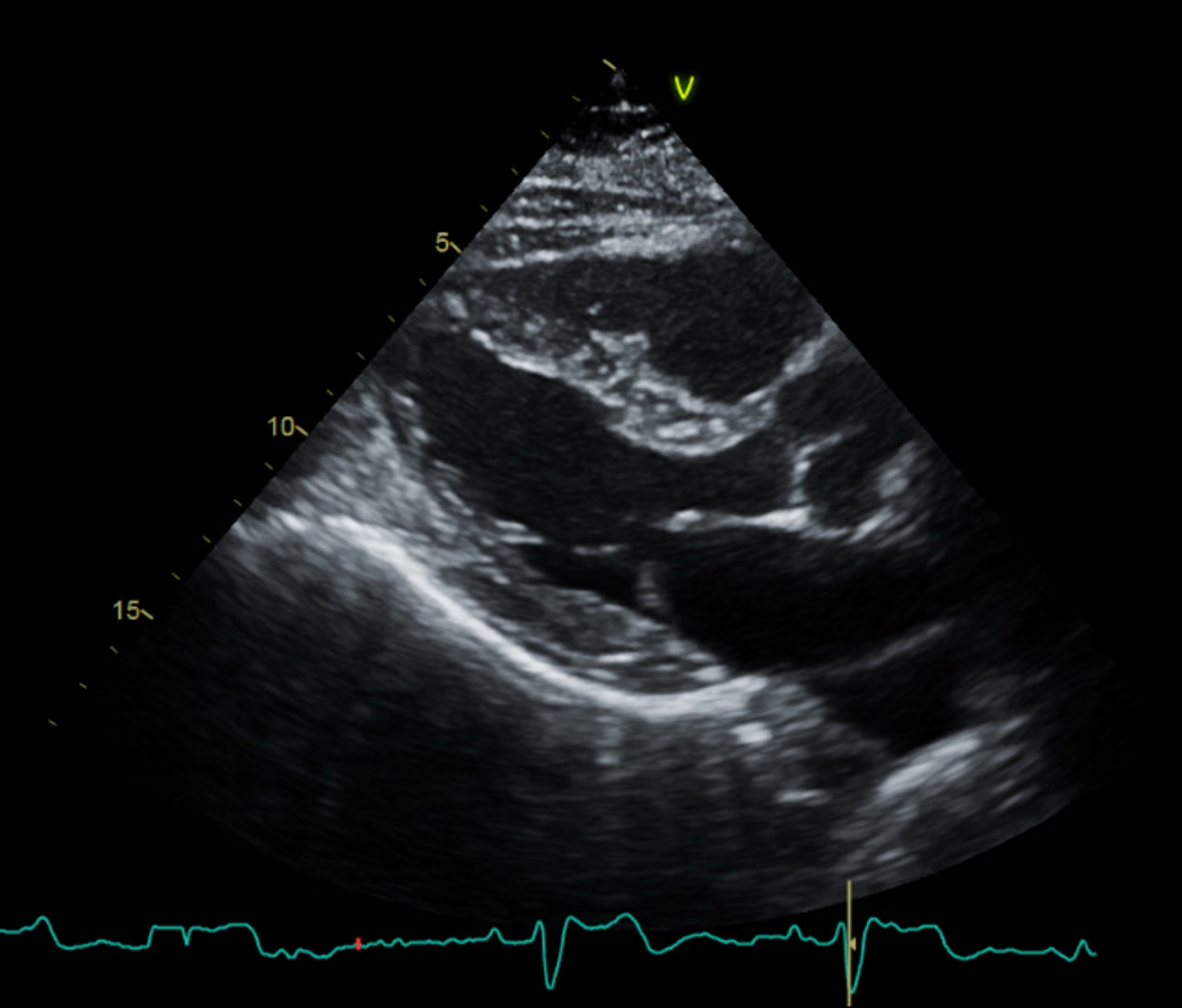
Parasternal long-axis view of the patient's baseline echocardiogram did not show pericardial effusion.
